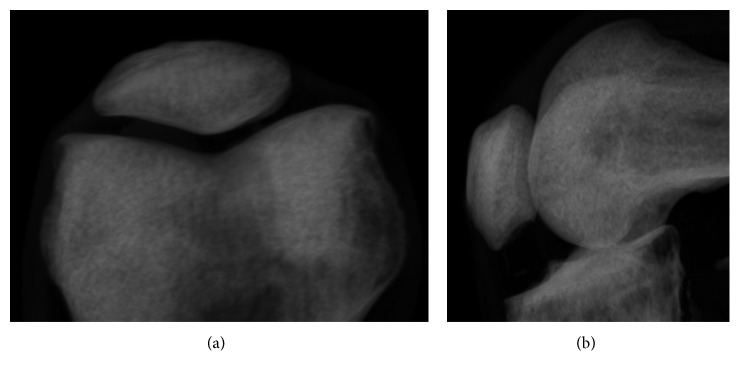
Pseudo-X-rays of a left knee created from a CT scan: (a) axial (skyline) and (b) sagittal (lateral).
